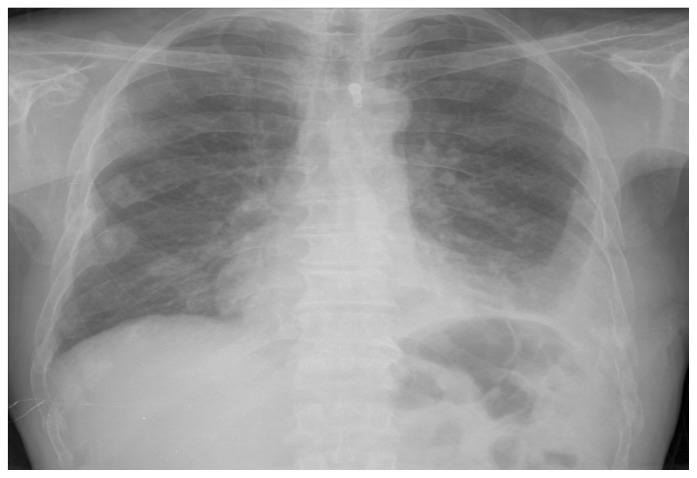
Chest radiograph showing pleural effusion on the left
